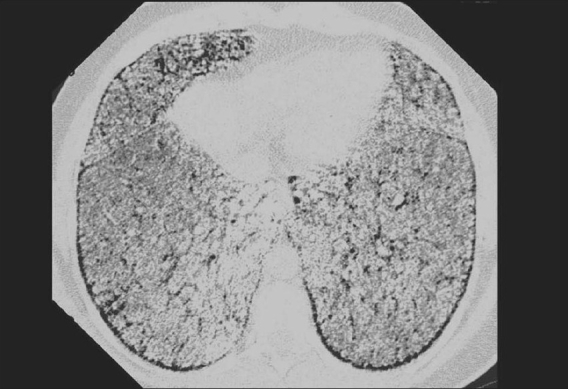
HRCT showing features of pulmonary alveolar microlithiasis. Note the innumerable calcific nodules bilaterally throughout both lungs giving rise to a sand-like appearance
